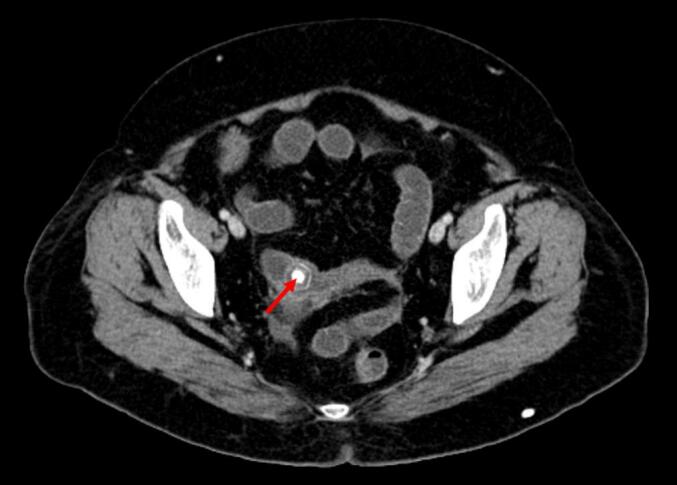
Axial abdominal CT scan view showing the stone in the intraluminal of the small bowel (red arrow). (For interpretation of the references to colour in this figure legend, the reader is referred to the web version of this article.)
